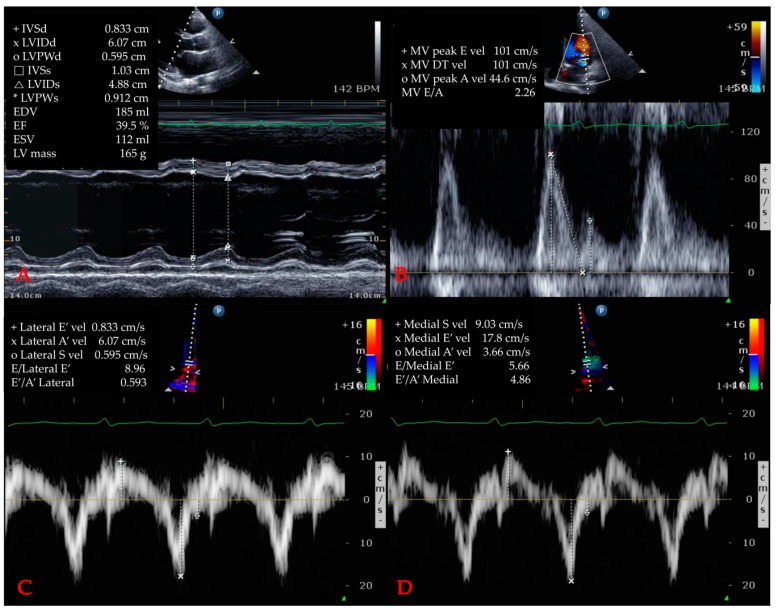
Example of echocardiography. (A) left ventricle ejection fraction measurement via Teichholz method. (B) measurement of mitral valve parameters. (C) measurement of E/e’ via tissue Doppler image (lateral). (D) measurement of E/e’ via tissue Doppler image (medial) Abbreviations: IVSd = interventricular septum (diastolic); LV = left ventricle; LVIDd = left ventricular internal diameter (diastolic); LVPWd = left ventricle posterior wall (diastolic); IVSs = interventricular septum (systolic); LVIDs = left ventricular internal diameter (systolic); LVPWs = left ventricle posterior wall (systolic); EDV = end-diastolic volume; EF = ejection fraction; ESV = end-systolic volume; MV = mitral valve; E = peak early diastolic transmitral flow; vel = velocity; DT = deceleration time; A = peak late diastolic transmitral flow velocity; E’ = peak early diastolic mitral annulus velocity; A’ = peak late diastolic mitral annulus velocity.
